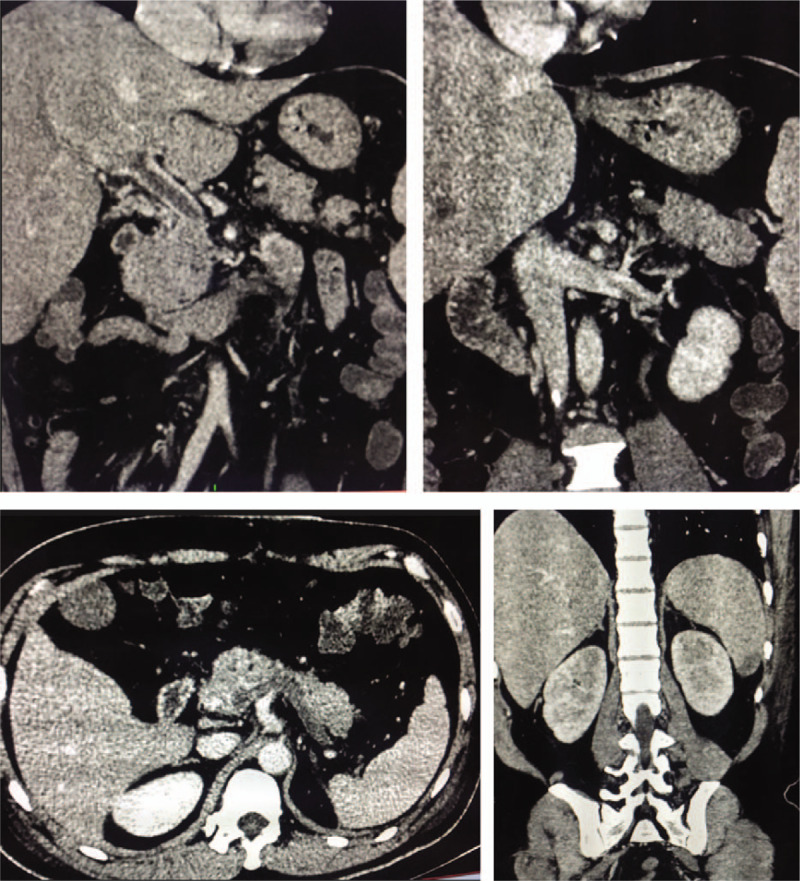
Results from abdominal CT scan with contrast: thrombosis of the portal vein, superior mesenteric vein, and injury of lower extremity of spleen. CT = computed tomography.
